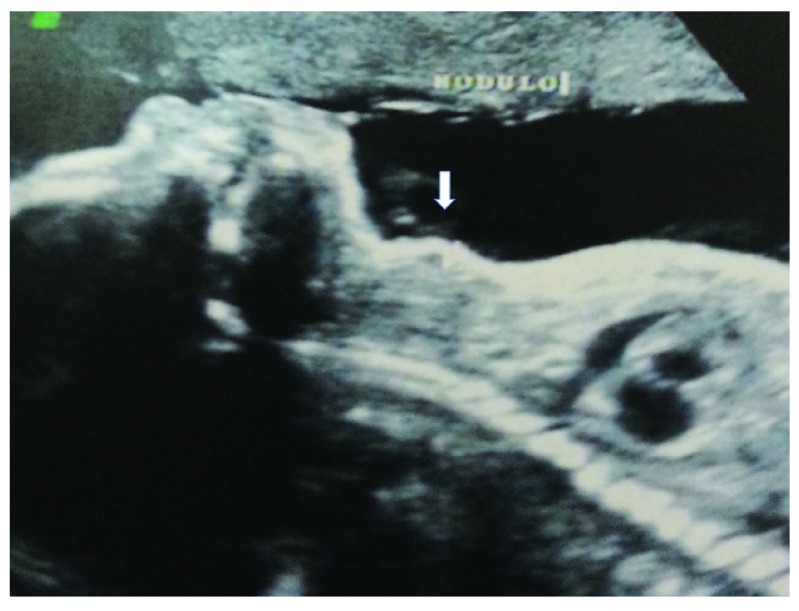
Prenatal ultrasound showing the neck mass.
